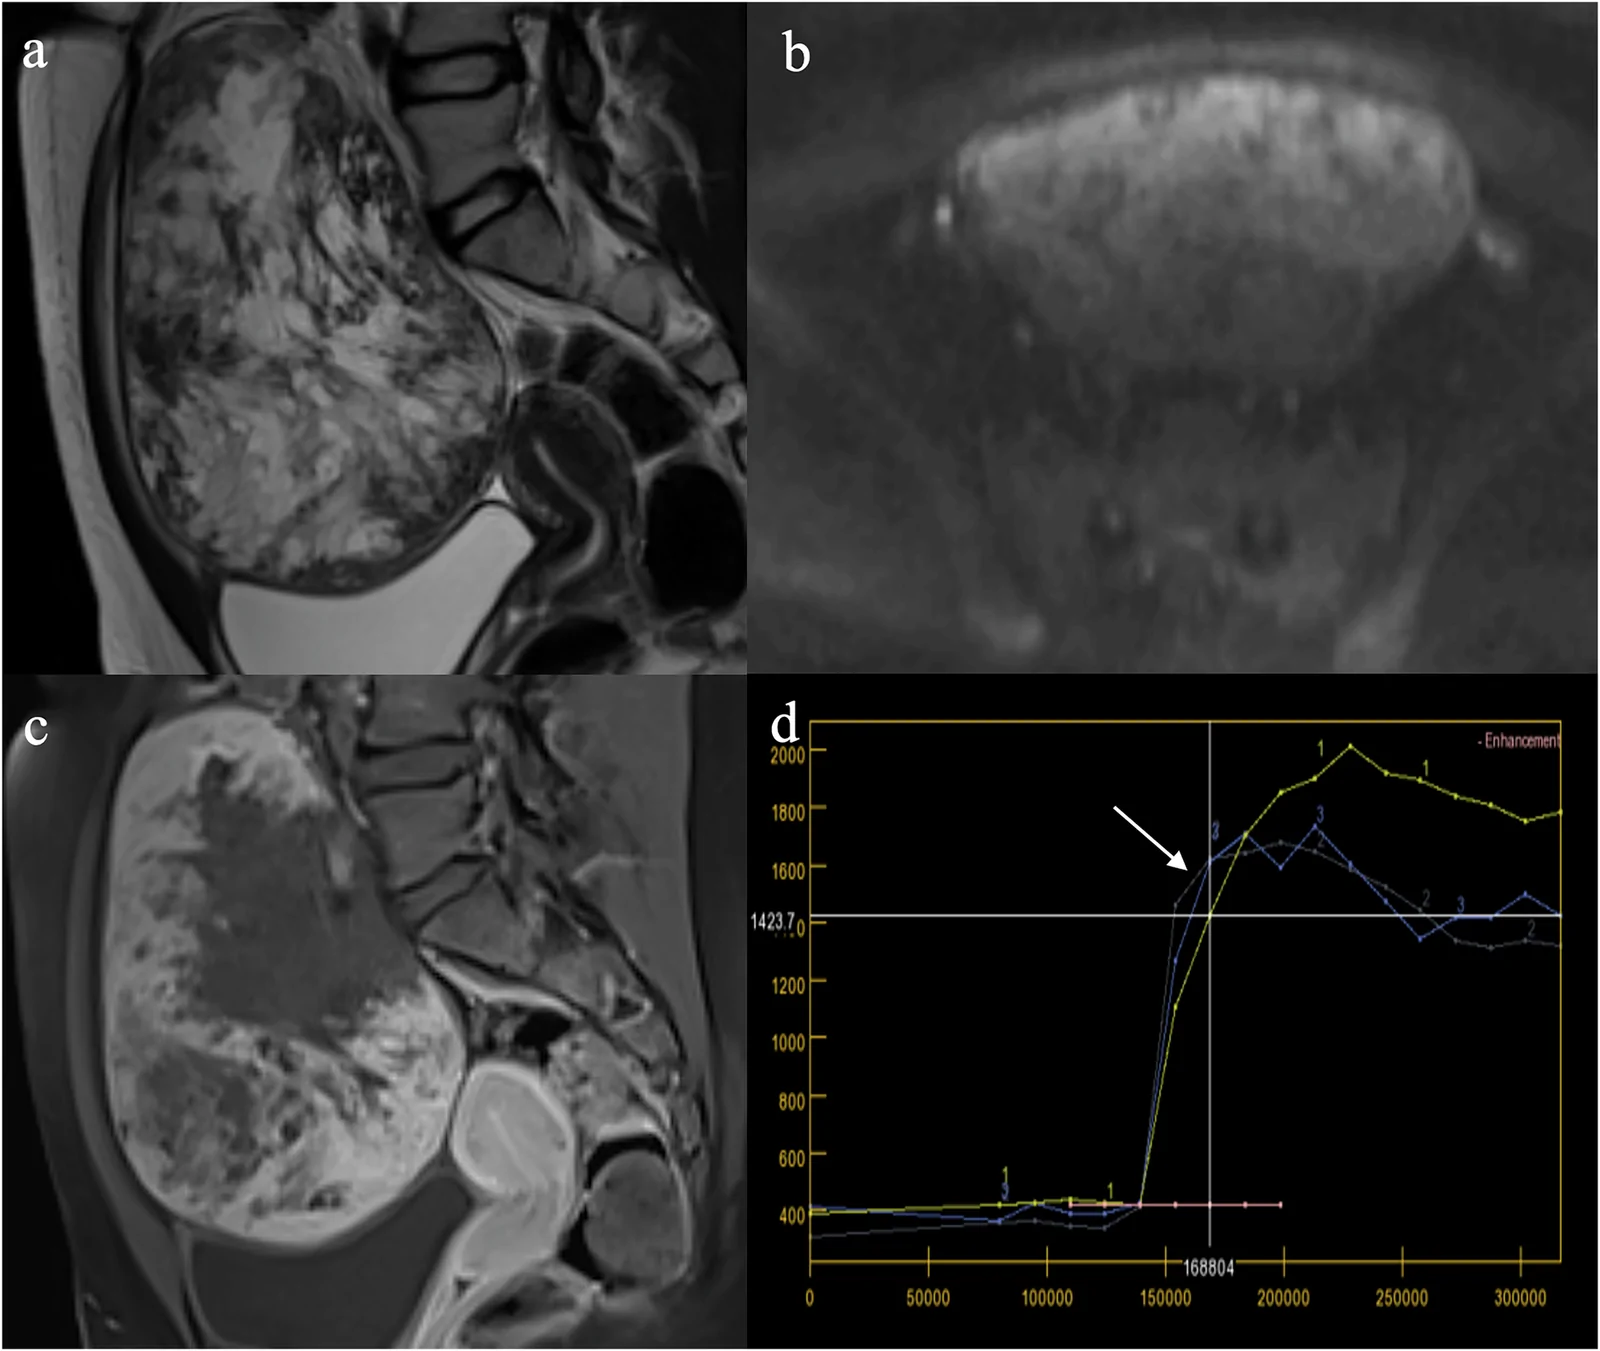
Example of benign O-RADS MR 5 tumor. a Sagittal T2-weighted sequence; b axial DWI sequence; c sagittal postcontrast fat-saturated T1-weighted sequence; d TIC from DCE MR sequence. A young patient (20 years old) is referred for left ovarian lesion characterization. The images show a large left ovarian tumor with heterogeneous signal intensity, including areas of high T2W signal, and high diffusion signal. Postcontrast imaging shows a large central necrotic area. TIC from DCE MR sequence shows a high-risk time-intensity curve (blue curve with white arrow) with a particular washout. Lesion is classified as O-RADS MR 5. Given the young age of the patient and the presence of a typical washout pattern on TIC, a sclerosing stromal tumor was suspected. Conservative surgery was performed, and pathology confirmed the diagnosis
